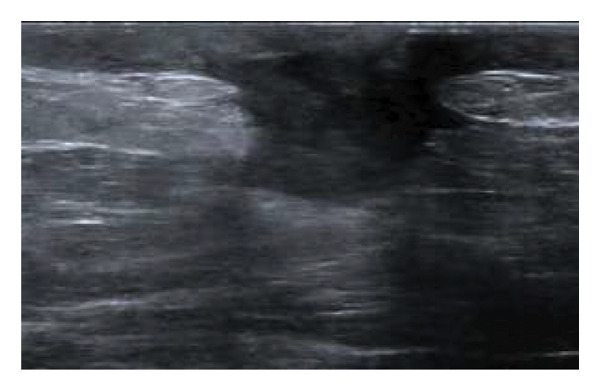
A 44‐year‐old patient presented with left breast mastodynia. Ultrasound demonstrates a hypoechoic nodular formation with irregular margins in the retro areolar region, inseparable from the areola–nipple complex, which appears markedly thickened (8). The Colour Doppler examination shows increased peripheral vascular signals (9).
